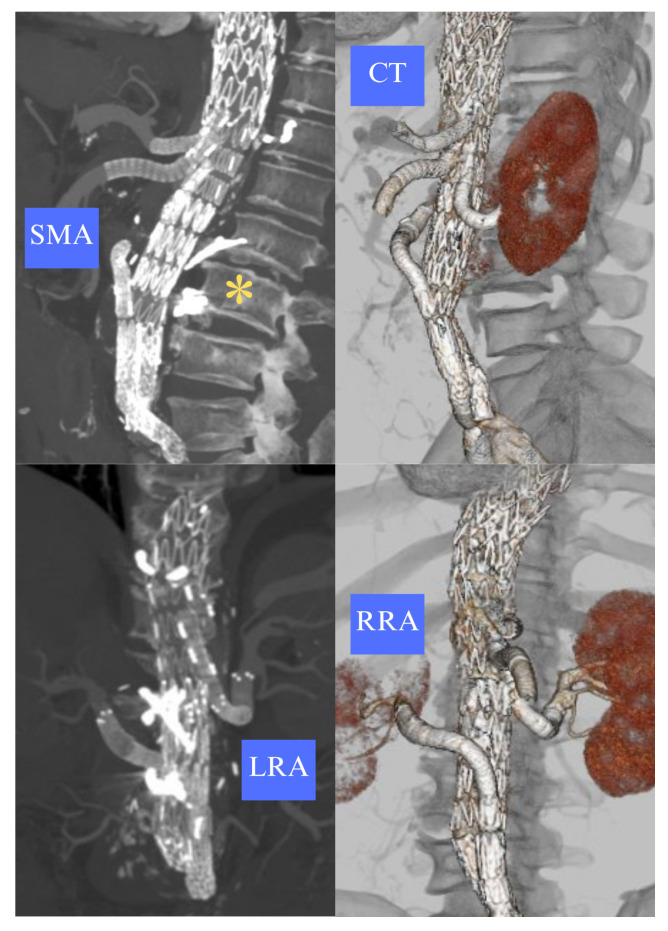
B-EVAR final result. MPR and 3D reconstructions from follow-up CTA showing complete expansion of the custom-made endograft with successful incorporation of CT, SMA, LRA and upward-oriented RRA. Segmental artery coil embolization is visible dorsally to the endograft (*).
